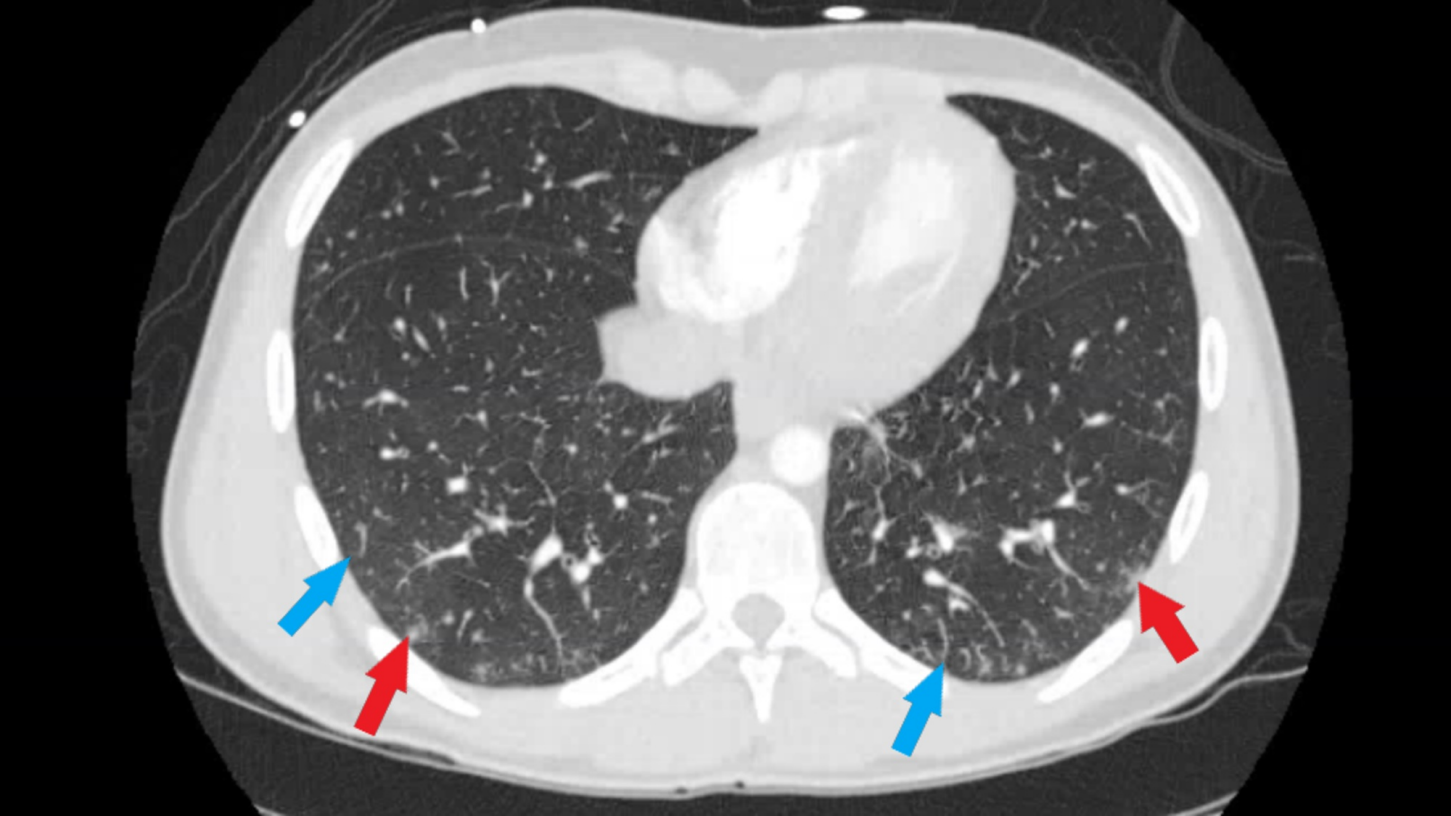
Computed tomography angiography (CTA) chest. CTA of the chest: Bilateral patchy ground-glass opacities (blue arrow), greatest at the posterior lower lobes. Some additional more nodular appearing opacities (red arrow) are present and also greater in the lower lobes.
